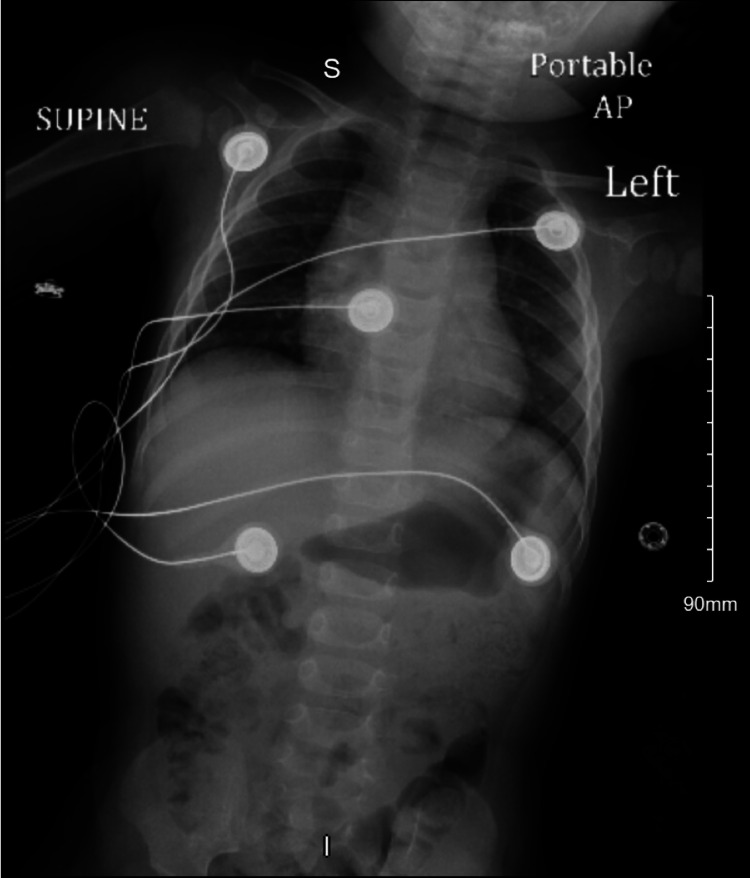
Chest X-ray demonstrating no acute pathology
